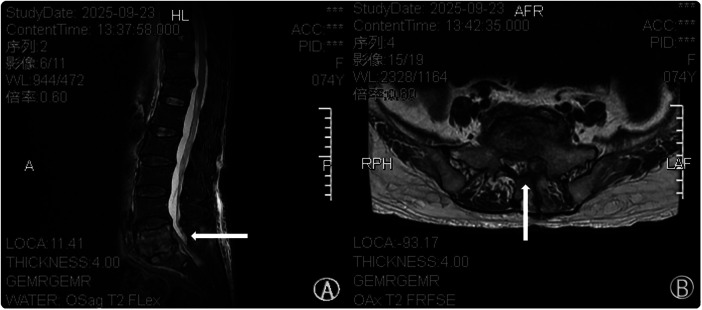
MRI showed a significant reduction in spinal abscess after more than a month of surgery.
